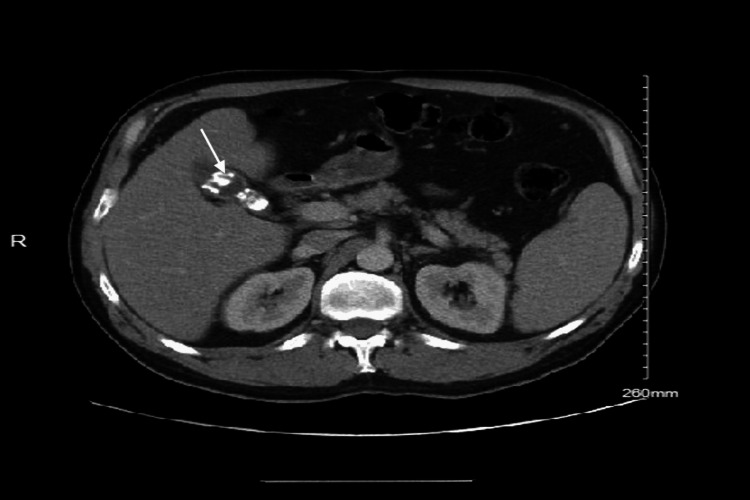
CT scan of the abdomen. The CT scan of the abdomen shows gallstones (white arrow) with the presence of fatty liver. There is no evidence of RUQ inflammation or bile duct dilation. CT: computed tomography, RUQ: right upper quadrant.
